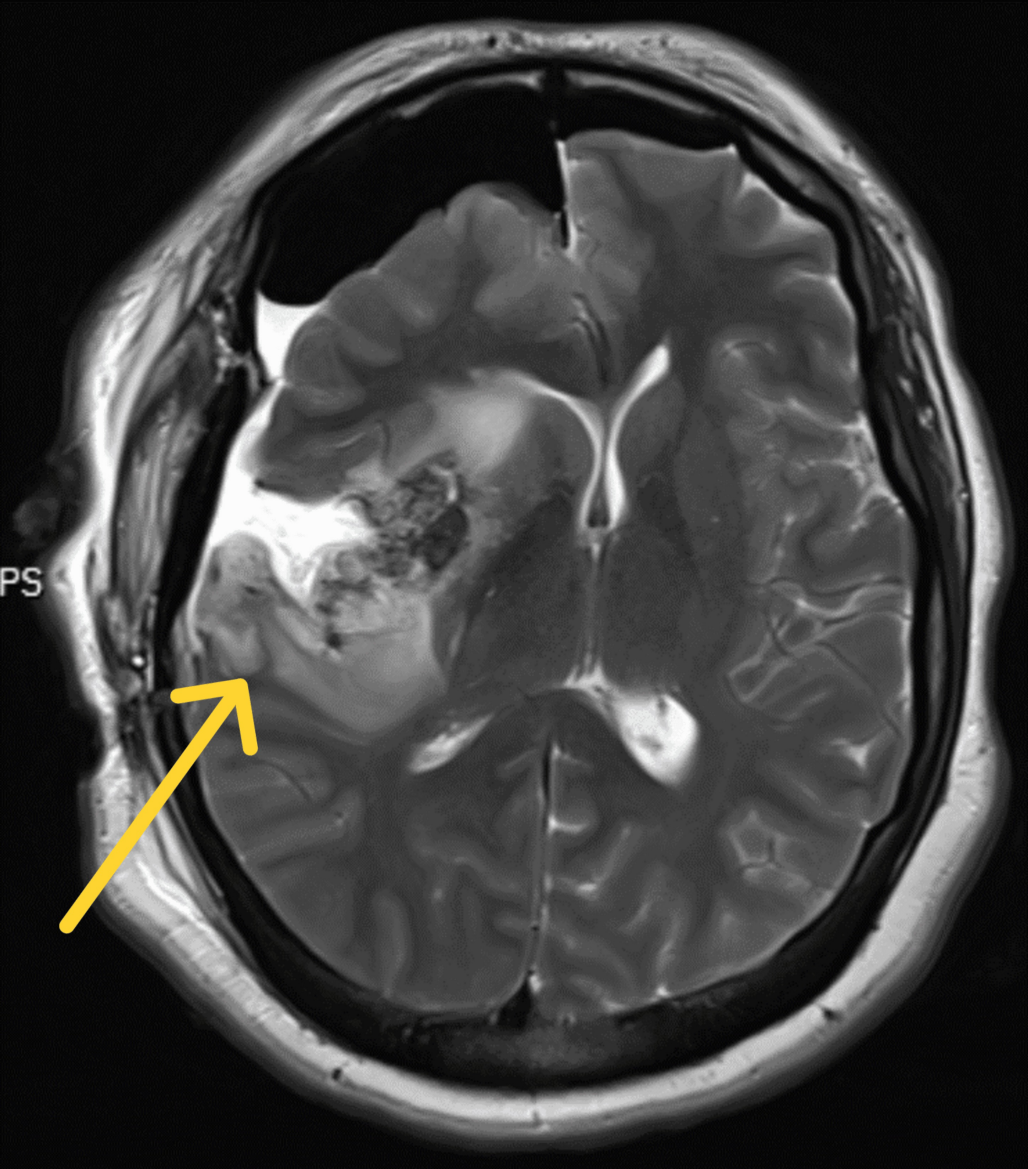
Postoperative MRI after second surgery (T2 axial view).
